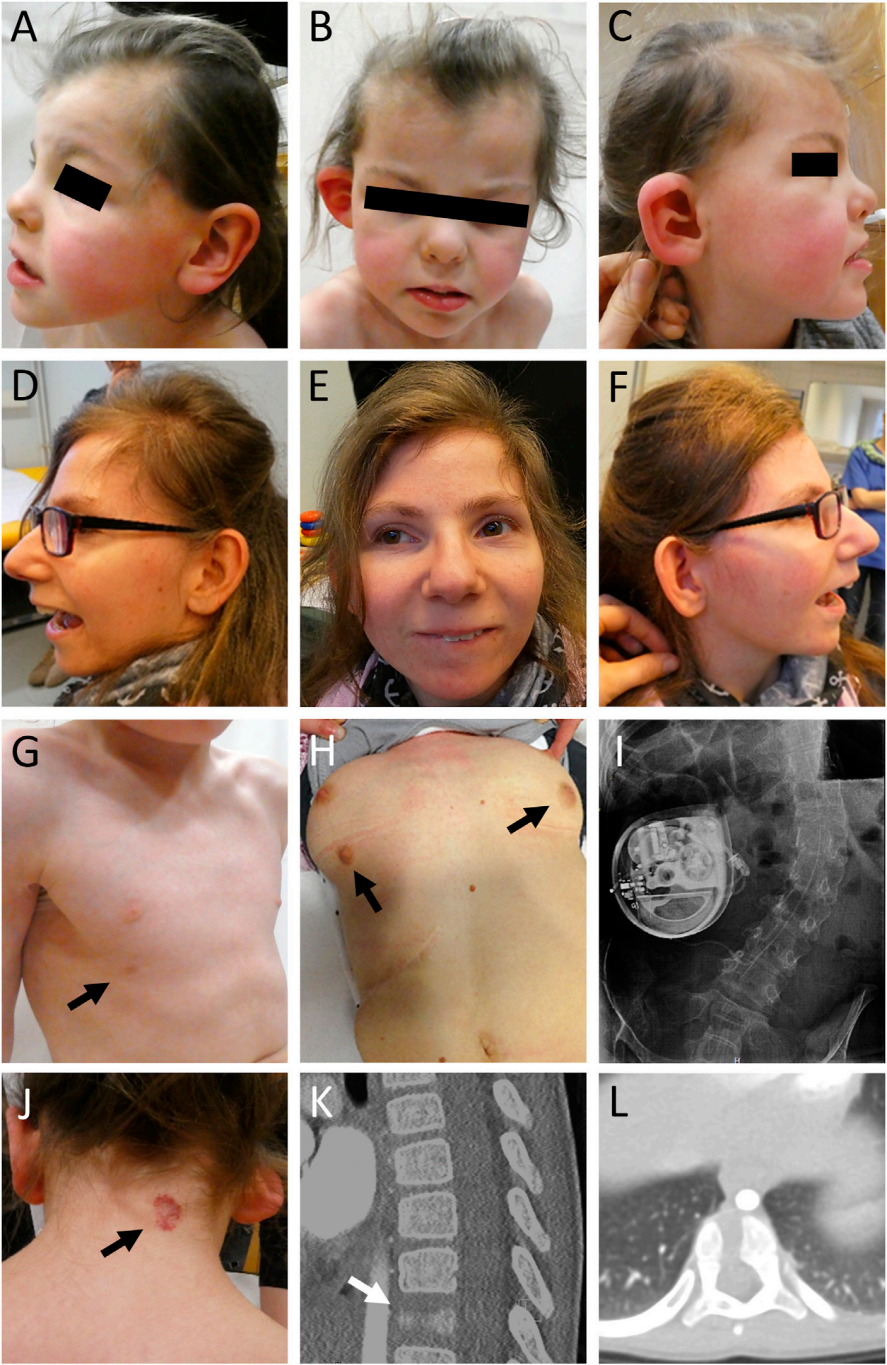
Clinical pictures and radiographs of affected individuals. (A–C) Clinical characteristics of individual 1 included microcephaly, facial asymmetry, widow’s peak, slightly low-set and posteriorly rotated ears, a protruding dysplastic right ear, prominent eyebrows, a wide nasal bridge, and a thin upper lip. (D–F) Individual 2 showed severe microcephaly, slightly low-set and posteriorly rotated ears, prominent eyebrows, strabismus, a prominent nose, a thin upper lip, and retrognathia. (G) Individual 1 showed a supernumerary nipple only on the right side (indicated by black arrow). (H) Individual 2 showed bilateral supernumerary nipples and breasts (indicated by black arrows) as well as (I) scoliosis. She received treatment for dystonia with a baclofen pump. (J) In addition, individual 1 presented with a low posterior hairline, a hemangioma (indicated by black arrow) on the neck, (K,L) and a butterfly vertebra Th11 (indicated by white arrow).
